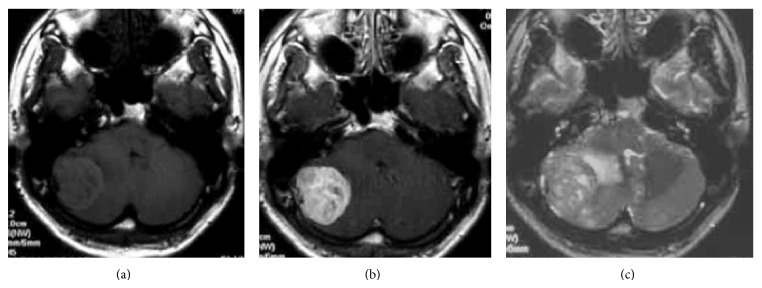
Preoperative T1-weighted plain (a), gadolinium-enhanced (b), and T2-weighted (c) magnetic resonance imaging showing the infratentorial extra-axial tumor with perifocal edema. Note that the tumor shows strong, homogeneous enhancement.
